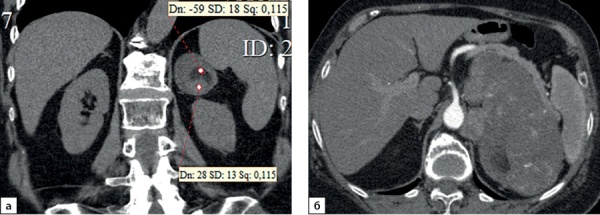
Рисунок 2. Компьютерные томограммы пациентки В., 70 лет с гигантской опухолью левого надпочечника (137х80 мм), деформирующая тело и хвост поджелудочной железы: а — изображение реформатированное в корональной плоскости, нативная фаза сканирования, в структуре мягкотканного образования с денситометрическими показателями до 28HU включения с низкой плотностью (до -59HU), соответствующие жировой ткани; б — артериальная фаза, включения жира не накапливают контрастный препарат.Figure 2. Computed tomography of patient V., 70 years old, with a giant tumor of the left adrenal gland (137x80 mm), deforming the body and tail of the pancreas: a — image reformatted in the coronal plane, native scanning phase, in the structure of the soft tissue formation with densitometric parameters up to 28HU inclusion with low density (up to -59HU), corresponding to adipose tissue; b — arterial phase, inclusions of fat do not accumulate a contrast agent.
